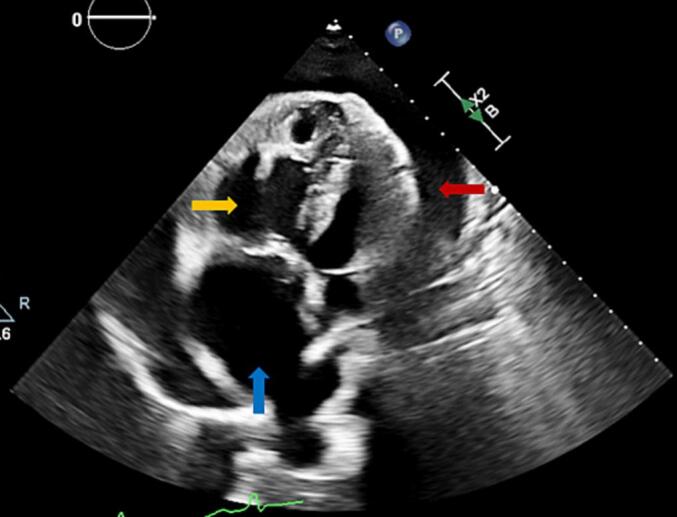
Transthoracic echocardiogram (TTE) obtained before initial pericardiocentesis. This figure shows TTE obtained on the day of presentation before the initial pericardiocentesis, revealing a large circumferential pericardial effusion (red arrows) severely dilated right atrium (blue arrow) and severely dilated right ventricle (yellow arrow).
